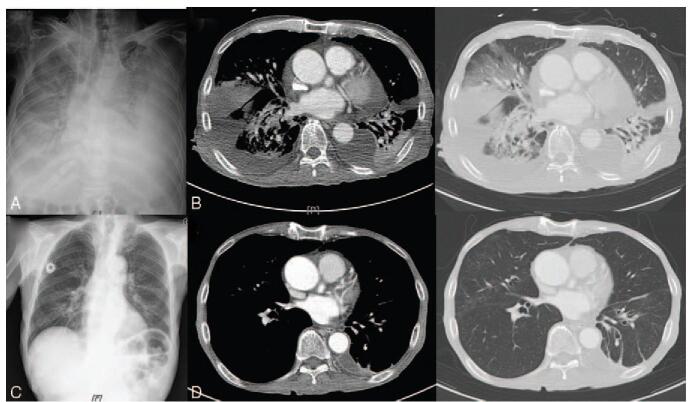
(A) Chest radiography demonstrated progressive patchy consolidations in the bilateral lung fields and bilateral pleural effusion. (B) Chest computed tomography (CT) scan shows encapsulated effusion surrounded by irregularly thickened pleura. (C) Chest radiography on 70th treatment day suggested improvement of patchy consolidations but remained atelectic. (D) Chest CT on 52nd treatment day revealed decreased extent of consolidations and patchy ground-glass opacity lesions in both lungs.
